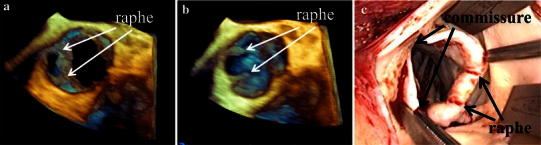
Real-time three-dimensional echocardiograms showed two commissures and two raphes on the fused area of both the right and non-coronary cusps in systole (a) and diastole (b). A photograph of the actual operation view shows two commissures and two raphes on the fused area of both the right and non coronary cusps (c)
